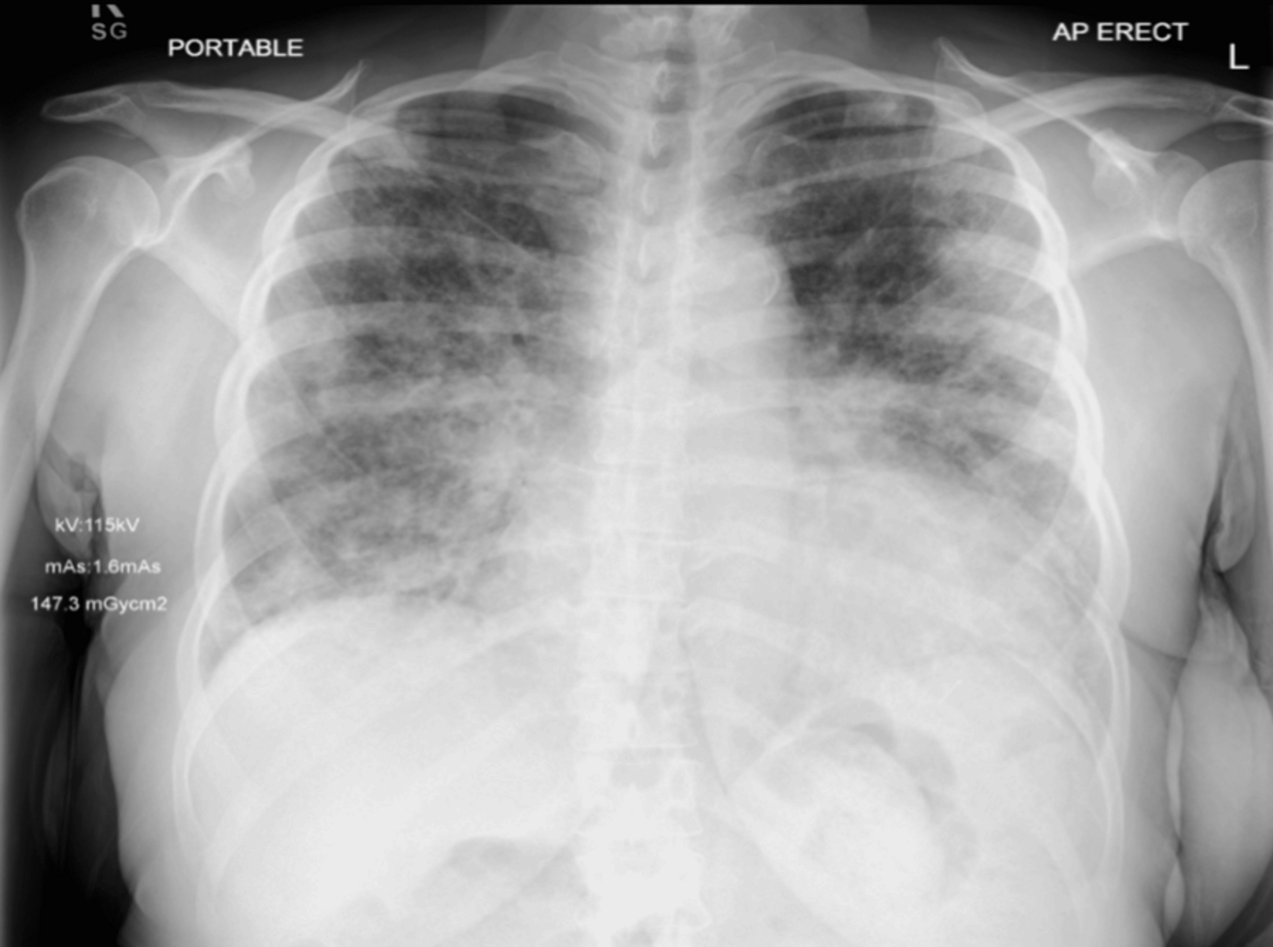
Chest X-ray demonstrating bilateral patchy and confluent opacities predominantly in the mid and lower lung zones, consistent with diffuse pulmonary edema—a radiographic feature of decompensated heart failure.
